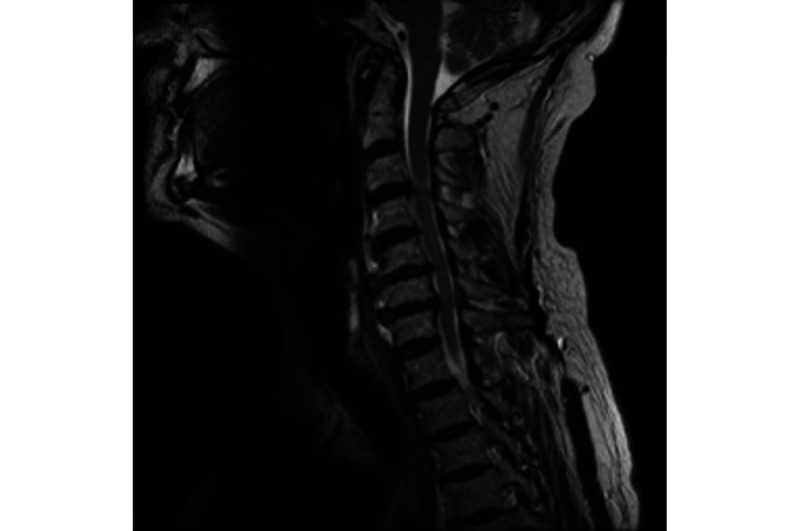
T2-weighted sagittal magnetic resonance imaging demonstrating multilevel degenerative cervical spondylosis and disc degeneration.
